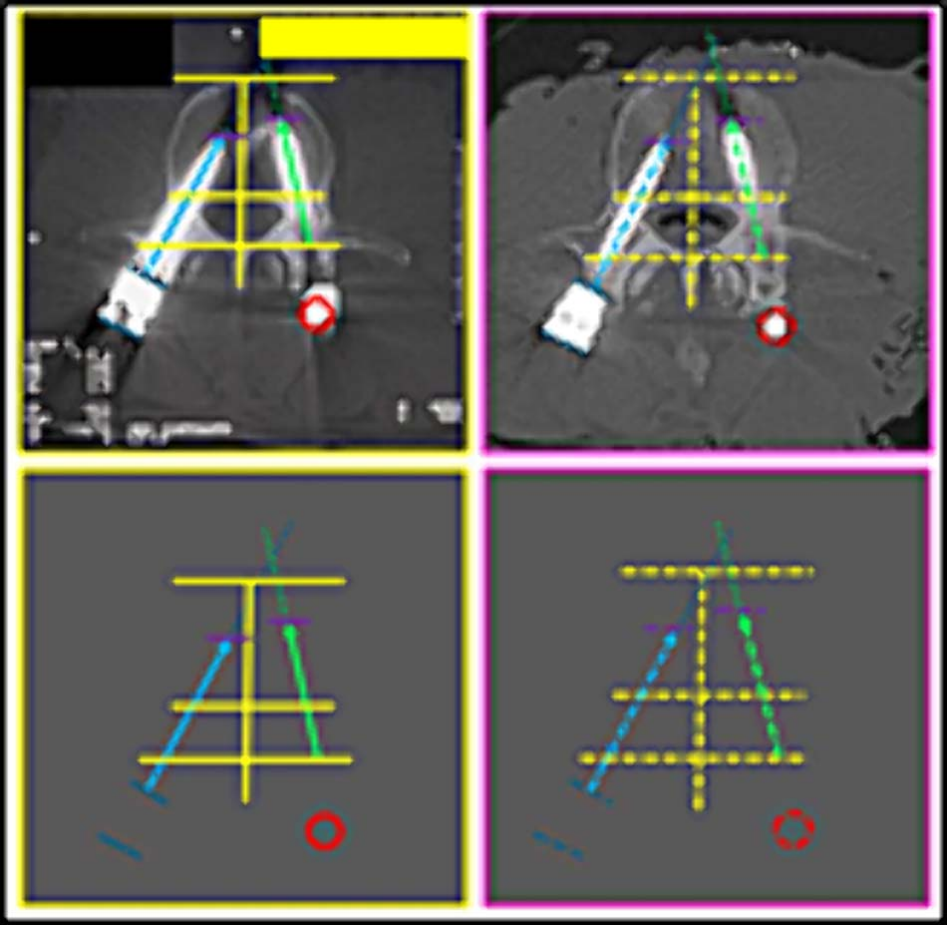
L3 vertebra. Upper panels, comparison between the axial slices of the antemortem (left) and postmortem (right) CT scans. Lower panels, respective concordant templates.
